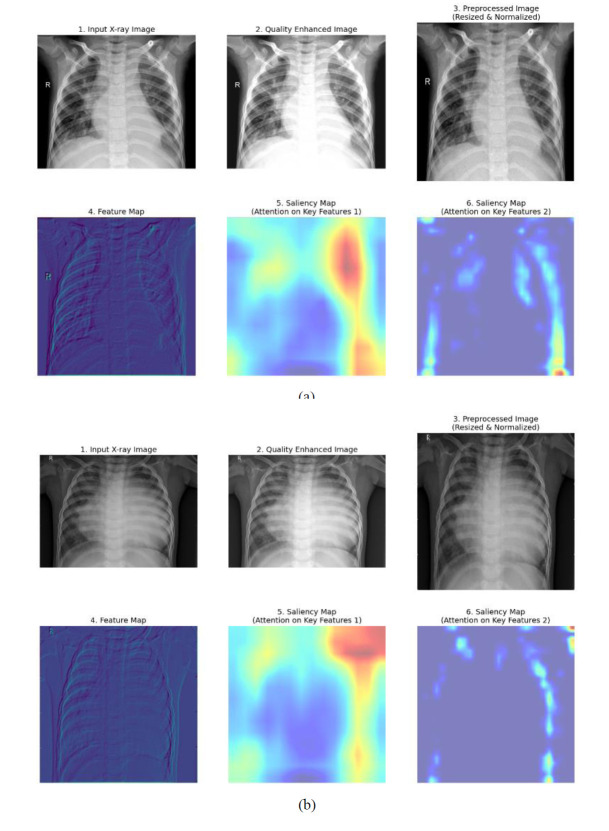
Sample input and output X-Ray images.
